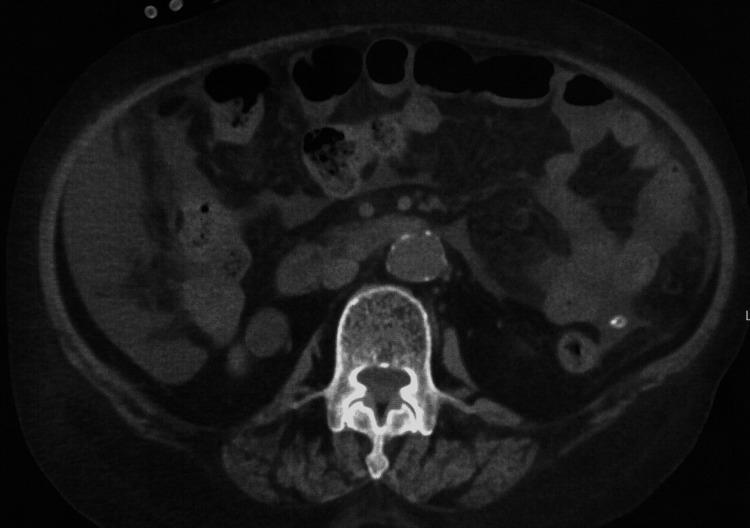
Computed tomography on admission showed no significant abnormalities other than the presence of a PD catheter and fluid. PD, peritoneal dialysis
